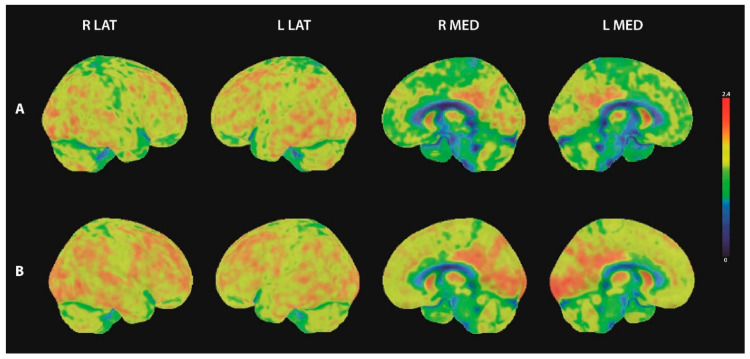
18F-FDG-PET images of the brain. Three-dimensional surface projections of FDG-uptake in lateral and medial view. (A) initial 18F-FDG-PET scan showed bilateral hypometabolism prefrontal medial and parietal, (B) follow up 18F-FDG-PET showed physiological cerebral FDG uptake. R lat = right lateral view; L lat = left lateral view; R med = right medial view; L med = left medial view.
